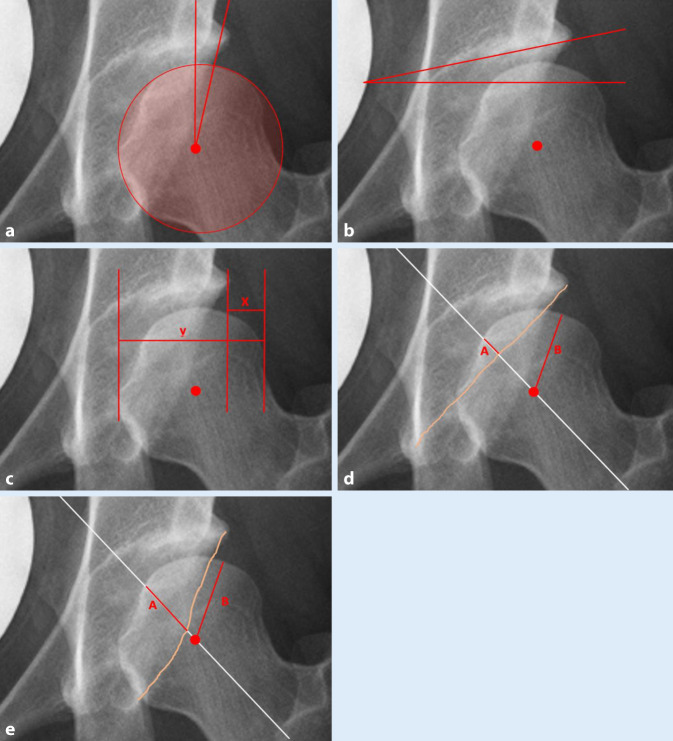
Anteroposterior (AP) pelvic radiographs of the radiological measurement in native pelvic X‑ray obtained in a standardized manner as previously described by Tannast et al. a Lateral center edge angle, b acetabular index, c extrusion index (x /y ×100), d anterior coverage (A /B ×100) and e posterior coverage (A /B ×100). *Red dot* femoral head center, *white line* axis of the femoral neck, *pink line* rim of the anterior or posterior acetabulum
